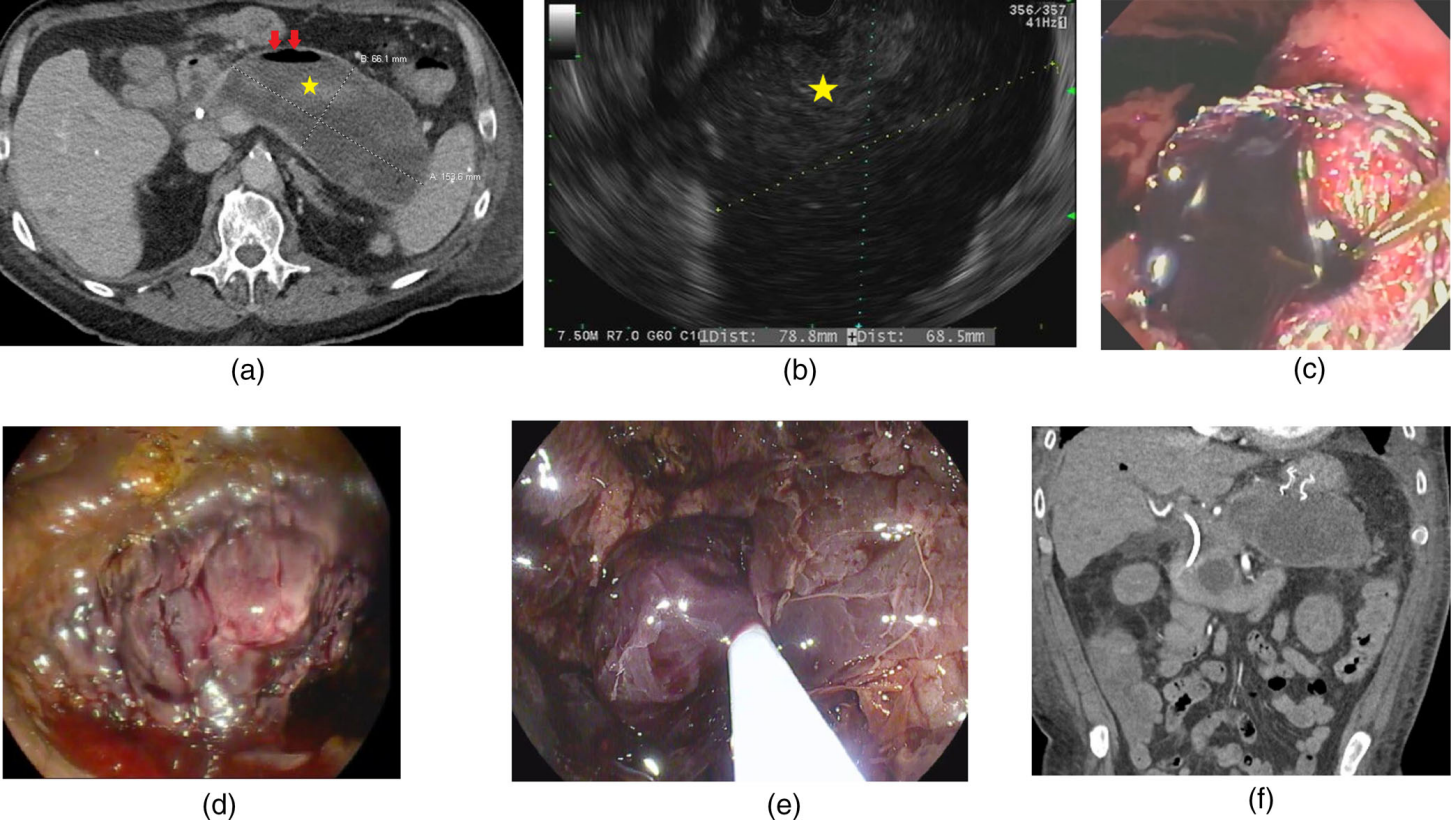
Endoscopic ultrasound‐guided drainage of hemorrhagic pancreatic fluid collection using a lumen‐apposing metal stent. (a) Computed tomography (CT) abdomen showing a large pancreatic fluid collection with hyperdense contents (asterisk) and air (red arrow) suggestive of infected hemorrhagic pancreatic fluid collection. (b) Endoscopic ultrasound showing a large pancreatic fluid collection with echogenic material (asterisk) suggestive of hemorrhage. (c) Endoscopic imaging showing blood draining from the cyst cavity after lumen apposing metal stent placement. (d) Endoscopic image showing blood clots inside the cyst cavity. (e) Endoscopic image showing removal of blood clots using a snare. (f) CT abdomen (coronal view) showing the appropriate position of lumen‐apposing metal stents (LAMS) inside the cyst cavity
